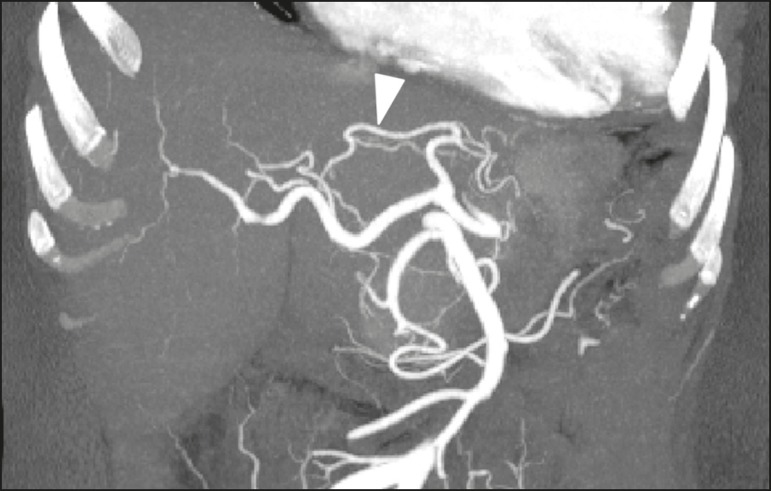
CT angiography reconstruction by maximum intensity projection
